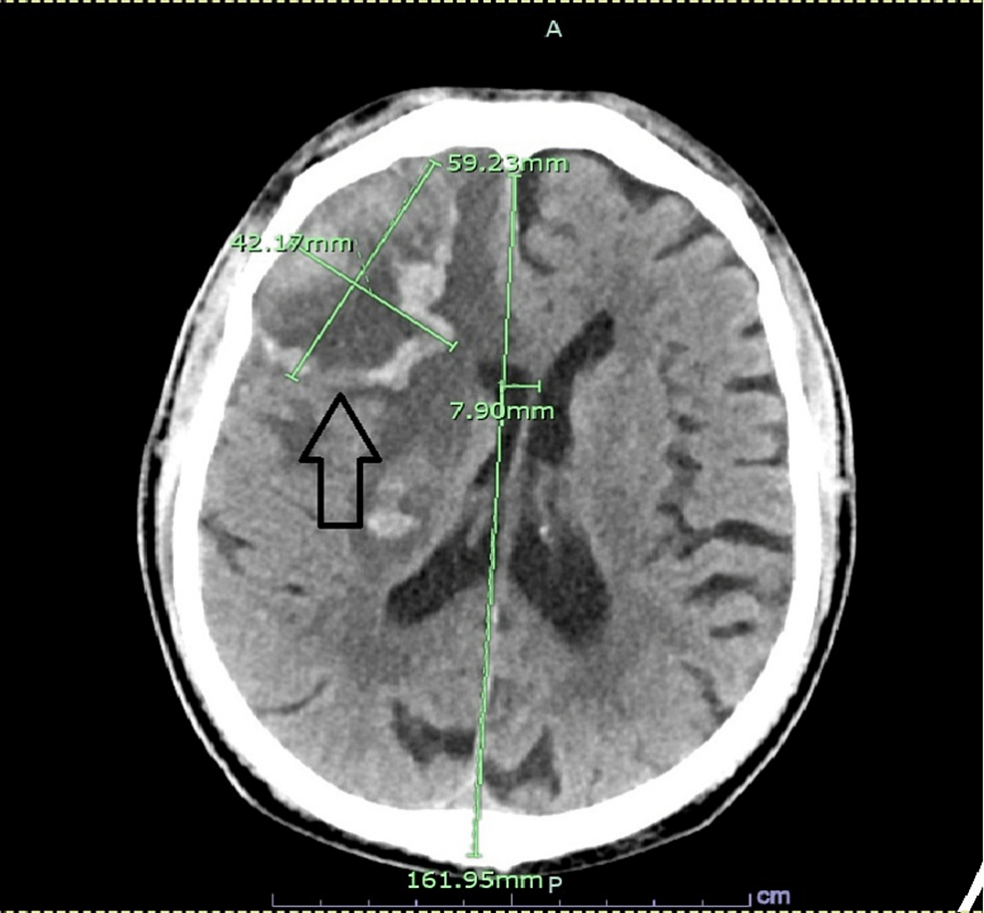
Cross-Sectional Computed Tomography (CT) Scan Head CT scan showing an irregularly shaped hemorrhagic mass in the right anterior frontal lobe with an 8 mm midline shift to the left (arrow)
